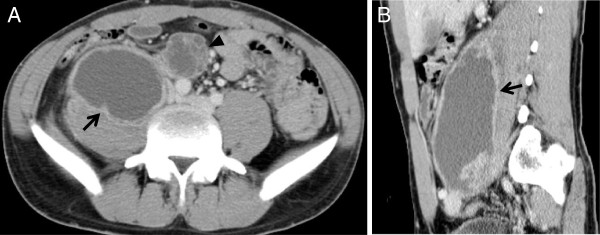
Contrast-enhanced computed tomography findings. Abdominal computed tomography shows a low-attenuation mass (arrow) with enhancing rim in the right psoas muscle. A para-aortic lymph node (arrowhead) adjacent to the psoas muscle is enlarged. (A) Axial view; (B) sagittal view.
